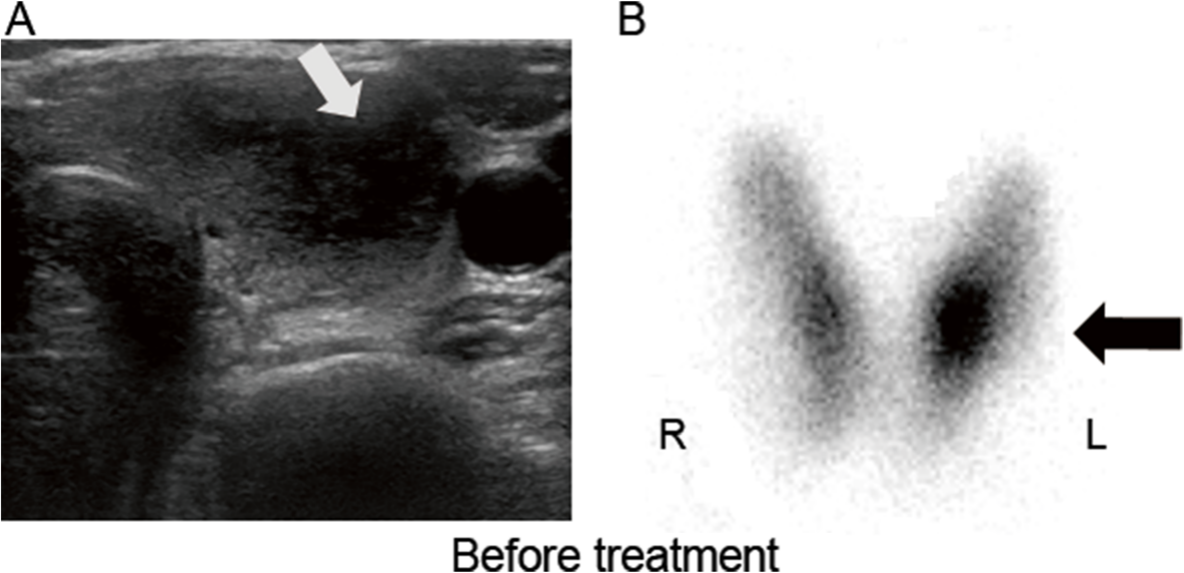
Initial thyroid ultrasound scanning and 99 m-Technetium scintiscan. A. Thyroid ultrasonography revealed a dyshomogeneous and hypoechoic nodule (15 × 0.8 mm) with an irregular and poorly defined border in the left thyroid lobe (indicated by a white arrow). B. Thyroid scintigraphy with 99 m-Tc showed a focal accumulation of radiotracer uptake in the lower lobe of the left thyroid, which represents the palpable tender nodule (indicated by a black arrow).
